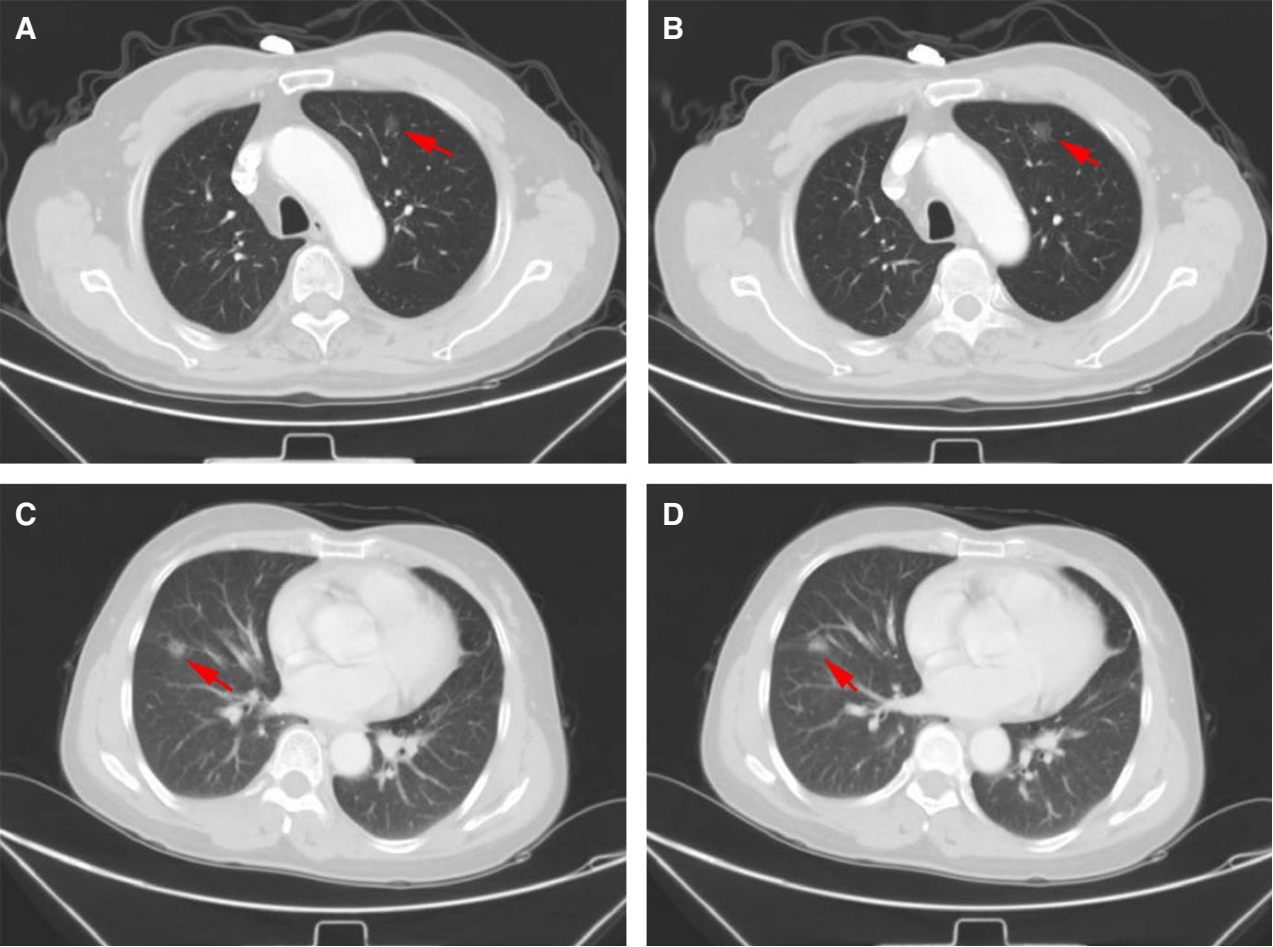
CT scans of the lung cancer patients. A, B, 1.3‐cm ground glass nodule was present in the left upper lobe of the lung. C, D, 1.0‐cm ground glass nodule was present in the right middle lobe of the lung
